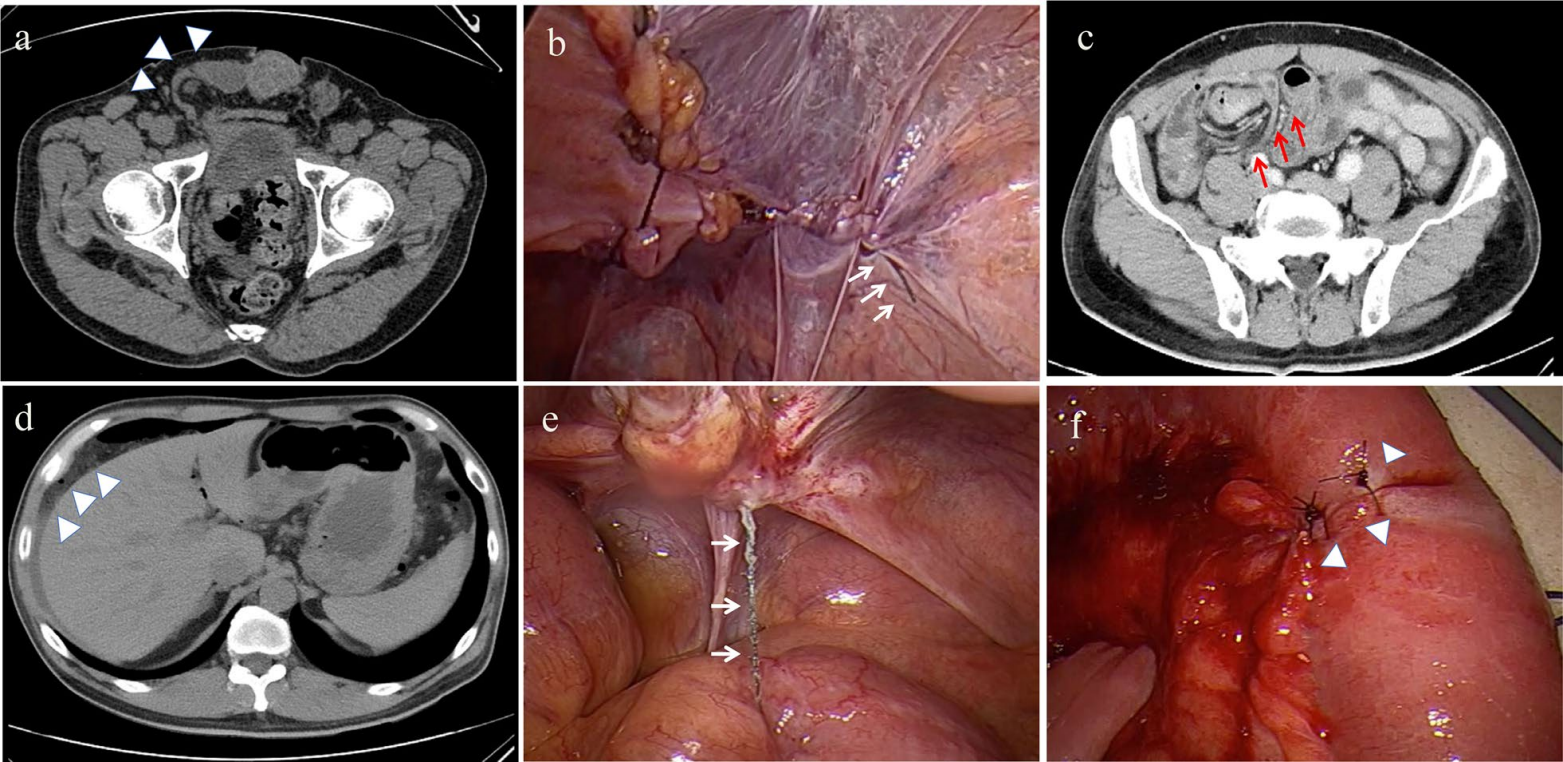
Preoperative and intraoperative images in Case 2. a The CT shows a right direct inguinal hernia in the prone position (white arrowheads). b The self-anchoring barbed suture device is used to close the right inguinal peritoneal defect from the left side to the right. The residual tail is less than 1 cm (white arrows). c The patient was readmitted with unremitting abdominal pain on postoperative day 10. There is obvious edema of small intestine, possibly volvulus of the ileum (red arrows). d Free air and ascites in the abdominal cavity, indicating perforation of the small intestine (white arrowheads). e The tail of the barbed suture is much longer and has inserted into the small intestine (white arrows). f After release of redundant barbed suture and removal of the tail of the barbed suture the perforated small intestine is closed with 2 stitches (white arrowheads)
